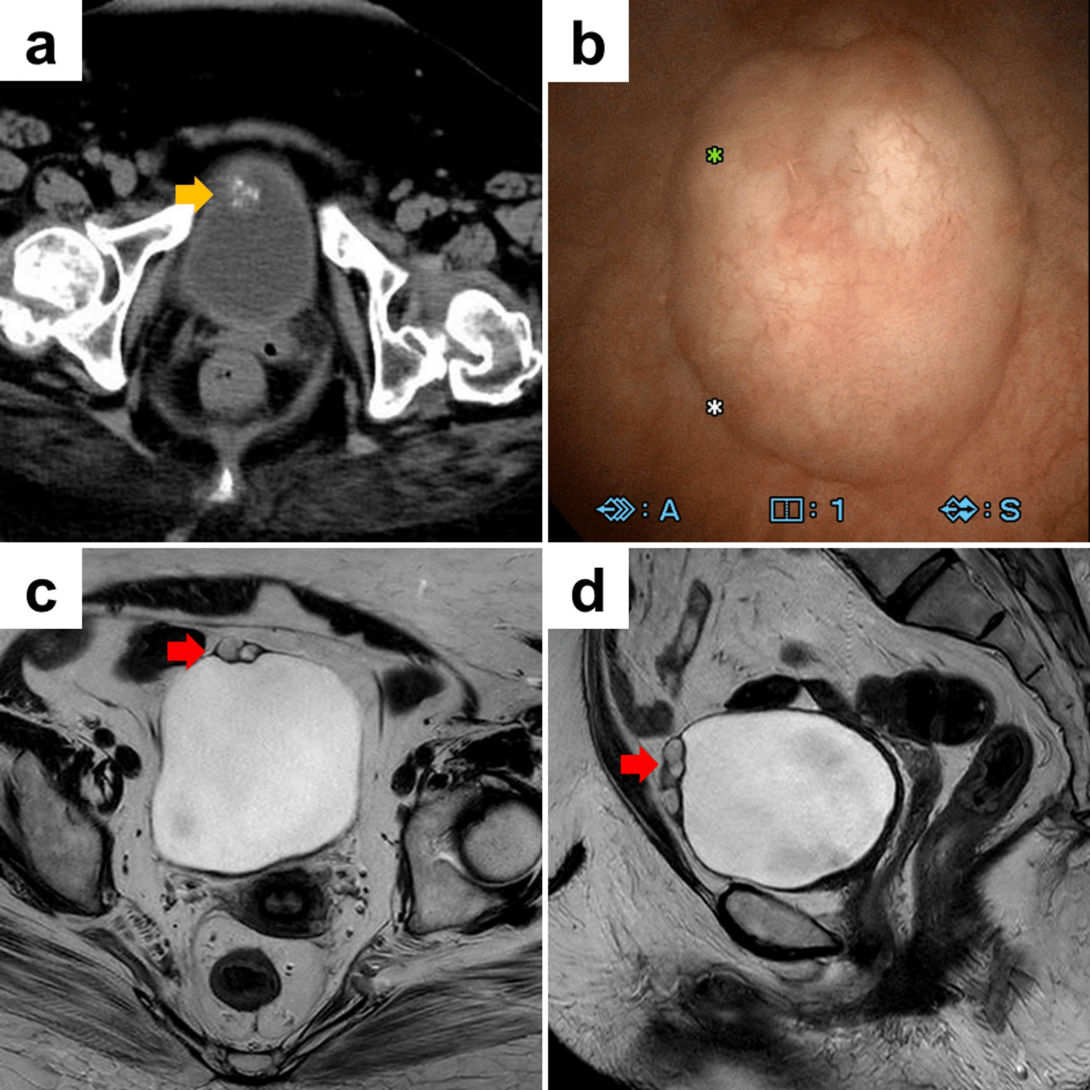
Imaging findings of the bladder tumor (a) Computed tomography (CT) shows the presence of a 2 cm-sized bladder tumor with calcification at the dome of the bladder. The yellow arrow indicates the presence of a bladder tumor. (b) Cystoscopy shows the presence of a bladder tumor at the dome. The mucosal surface of the tumor was observed to be unremarkable. (c) and (d) T2-weighted magnetic resonance imaging shows the presence of a 2 cm-sized multifocal cystic lesion in the anterior wall of the dome of the bladder (c: axial section; d: coronal section).
